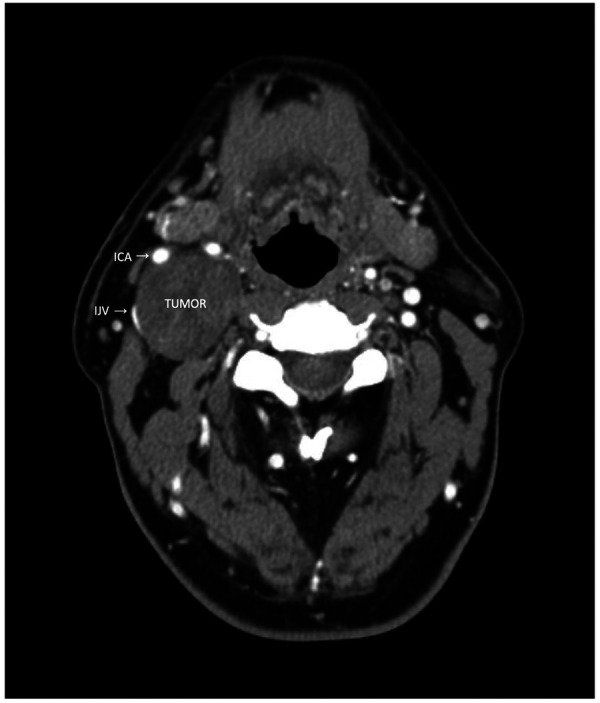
Misclassified case based on vessel displacement pattern. Axial contrast-enhanced CT image of the neck shows separation of the internal carotid artery (ICA) and internal jugular vein (IJV), initially suggesting vagal nerve origin. However, intraoperative findings demonstrated sympathetic chain origin, and the patient developed postoperative Horner's syndrome.
